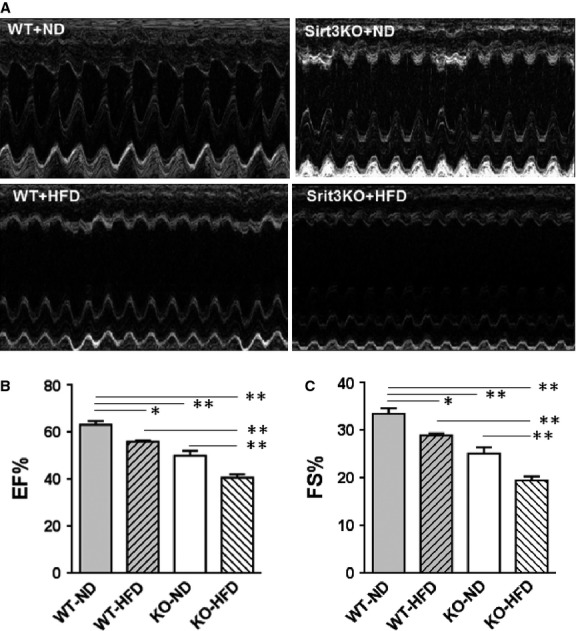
Assessment of cardiac function by echocardiography. (A) Representative M-mode tracings are shown. (B) Ejection fraction and (C) fractional shortening were determined. Values are means ± SEM, *n* = 10. *P*_I_ = *ns*, *P*_D_ and *P*_S_ ≤ 0.01; P* ≤ 0.05; *P* ≤ 0.01.
